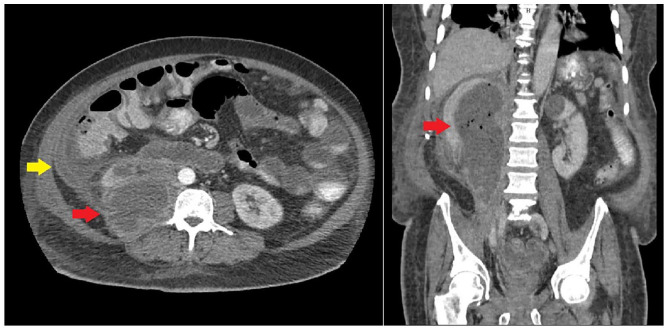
Repeat CT on hospital Day 7 showing improvement of renal abscess (red arrow)
